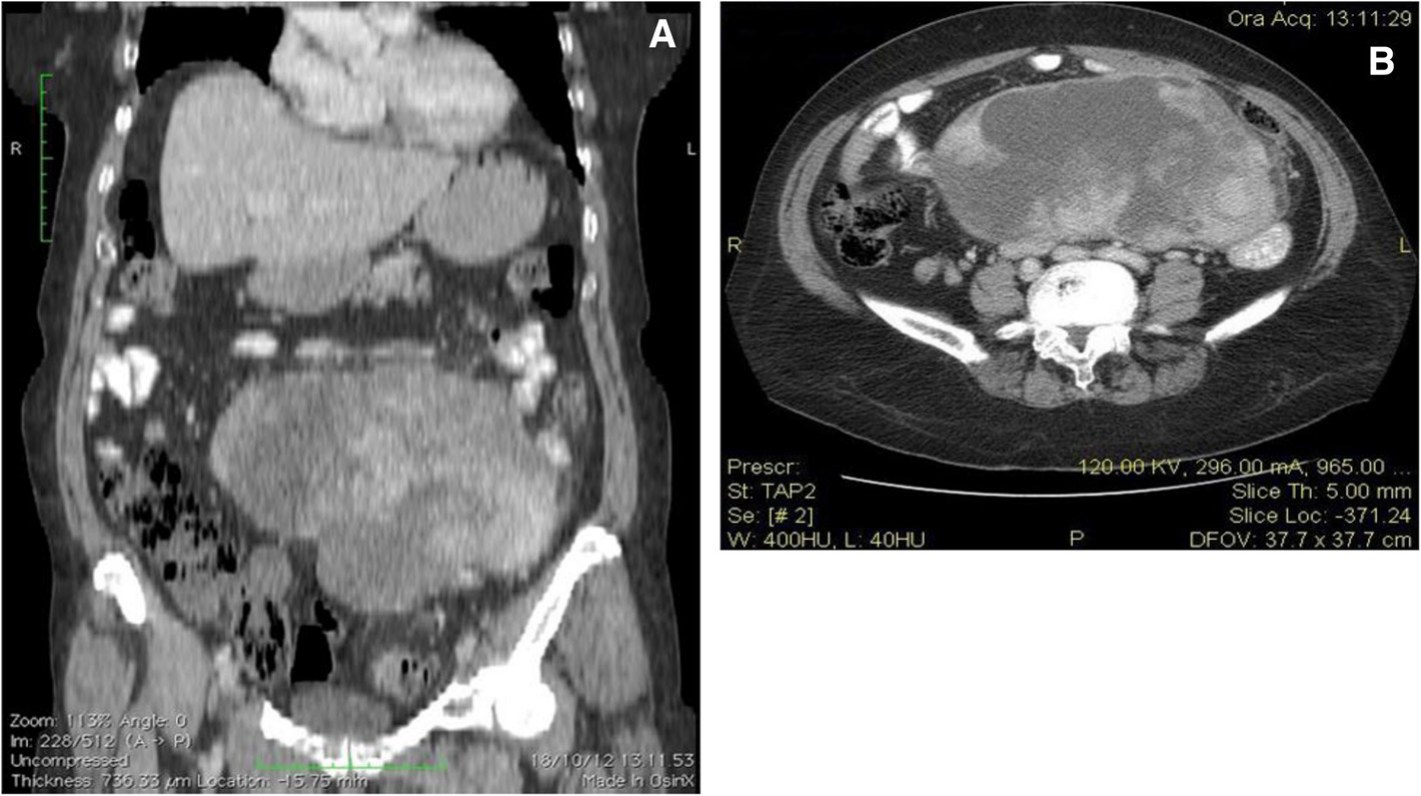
CT scans. (A) Coronal CT image demonstrating the extent of the mass; and (B) CT slice showing the heterogeneous density of the mass with lateral displacement of the bowel.
